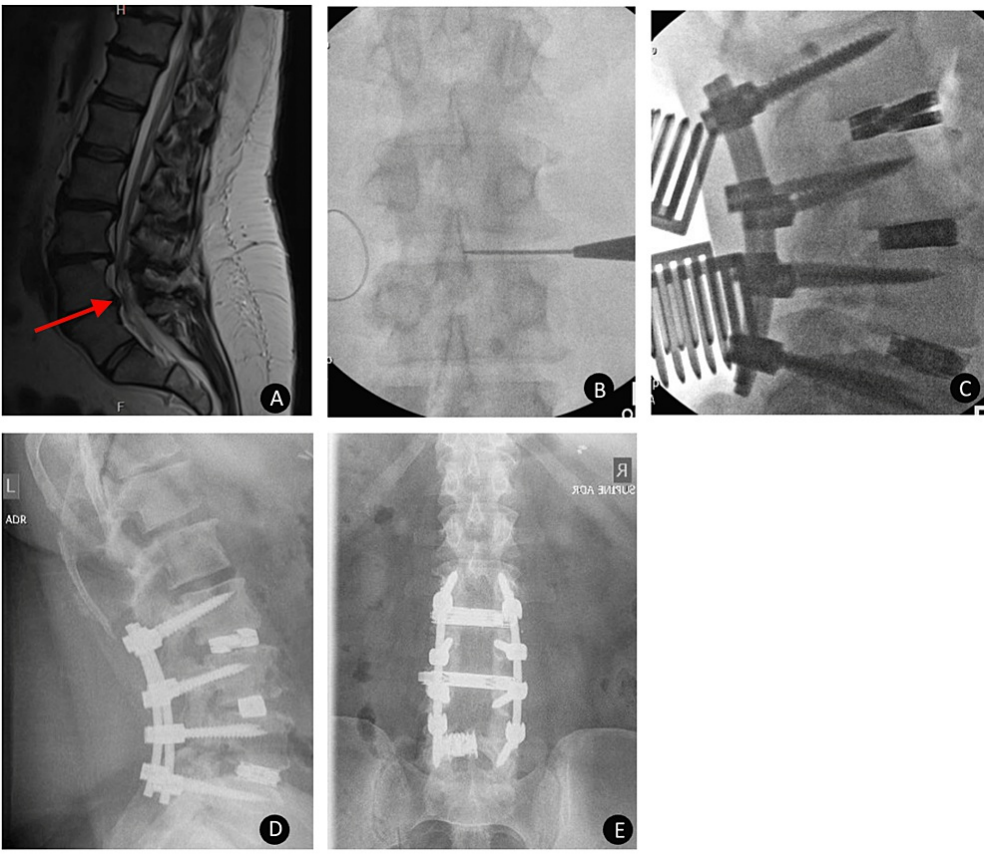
Case 5 postoperative radiographic evaluation of dual expandable spacer and screw placement A 53-year-old male with radiculopathy, neurogenic claudication, and low back pain. Preoperative sagittal MRI (A) showing moderate degenerative disc and facet disease at L3-S1 with moderate spinal stenosis (red arrow) at L4-S1 related to disk bulges, short pedicles, and prominent epidural fat. Intraoperative AP (B) and lateral (C) x-rays showing instrumentation and hardware placement along the lumbar spine. Postoperative lateral (D) and AP (E) x-ray images show intact interbody spacers without evidence of subsidence.
